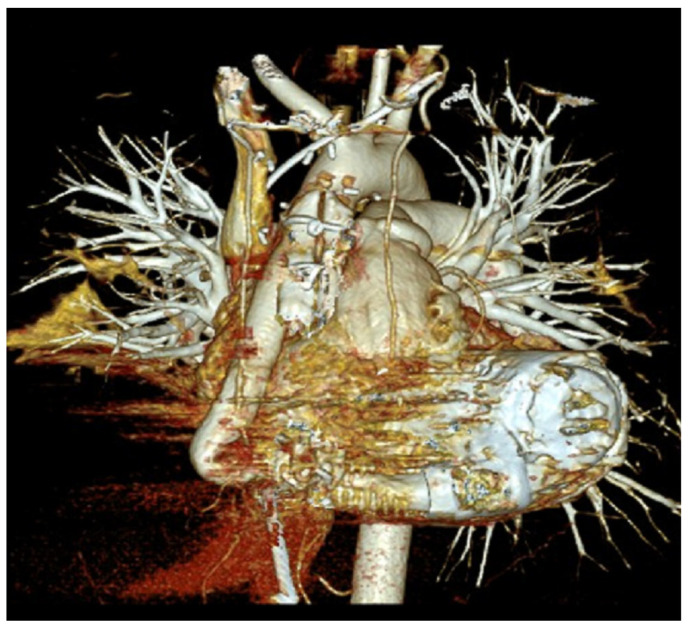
3D computed tomography reconstruction (PA view) of the heart and big vessels along with the LVAD system with its associated components in a patient with an implanted LVAD.
